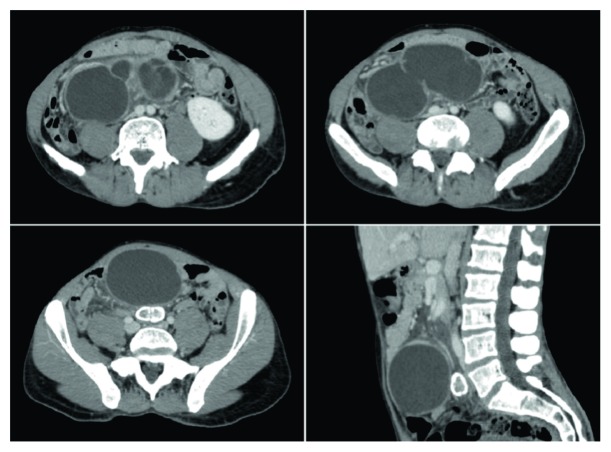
BMPM on CT scan. Contrast-enhanced CT scan shows the presence of 3 contiguous, communicating, and thin-walled cystic formations located at the level of the mesentery. The mass with larger dimensions is the most ventral one, and it is localized in contiguity with the abdominal wall, in the subumbilical region.
